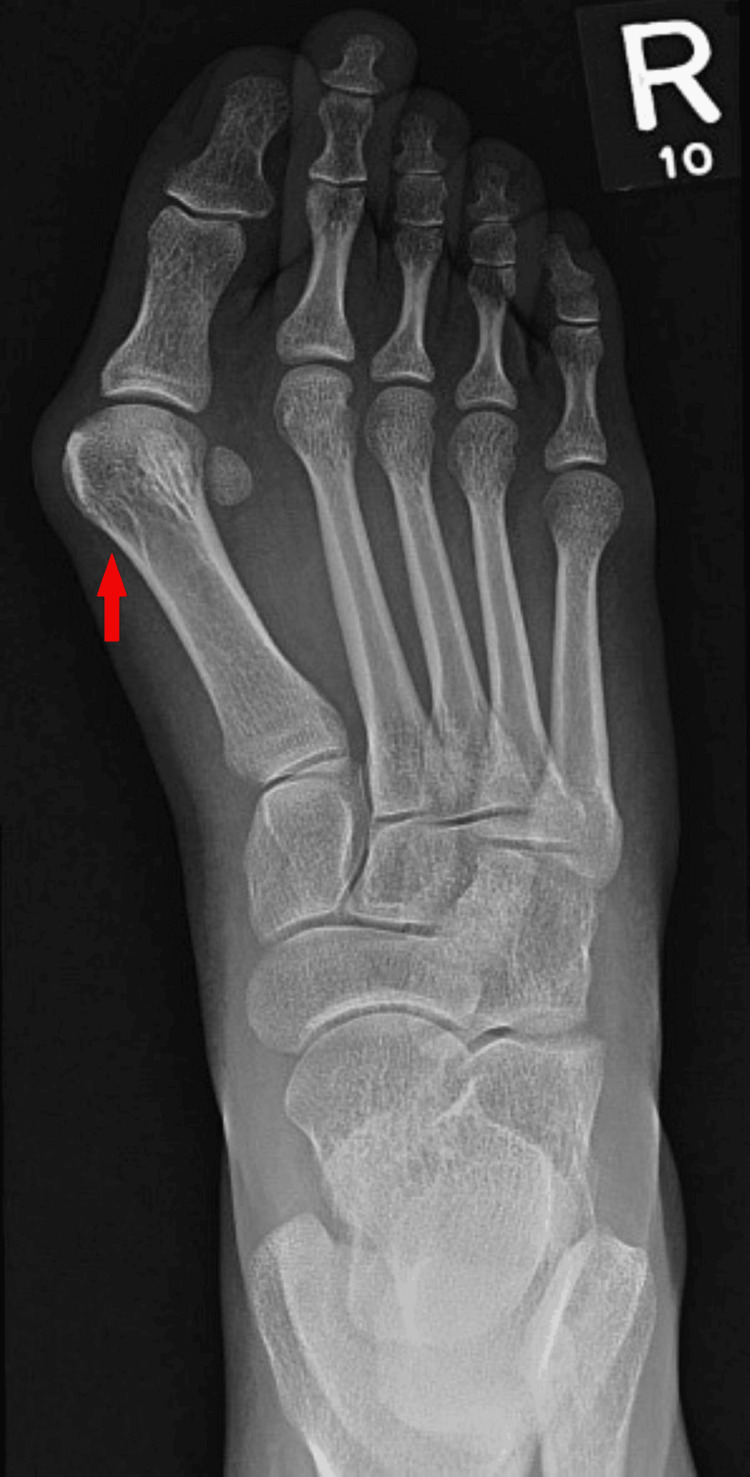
Right foot radiograph demonstrating severe hallux valgus deformity. Courtesy of Hani M Al Salam, Radiopaedia.org, rID: 12207. Adapted from Hani M Al Salam, Radiopaedia.org, available under the CC BY 4.0 International license (https://creativecommons.org/licenses/by/4.0/) [2].
